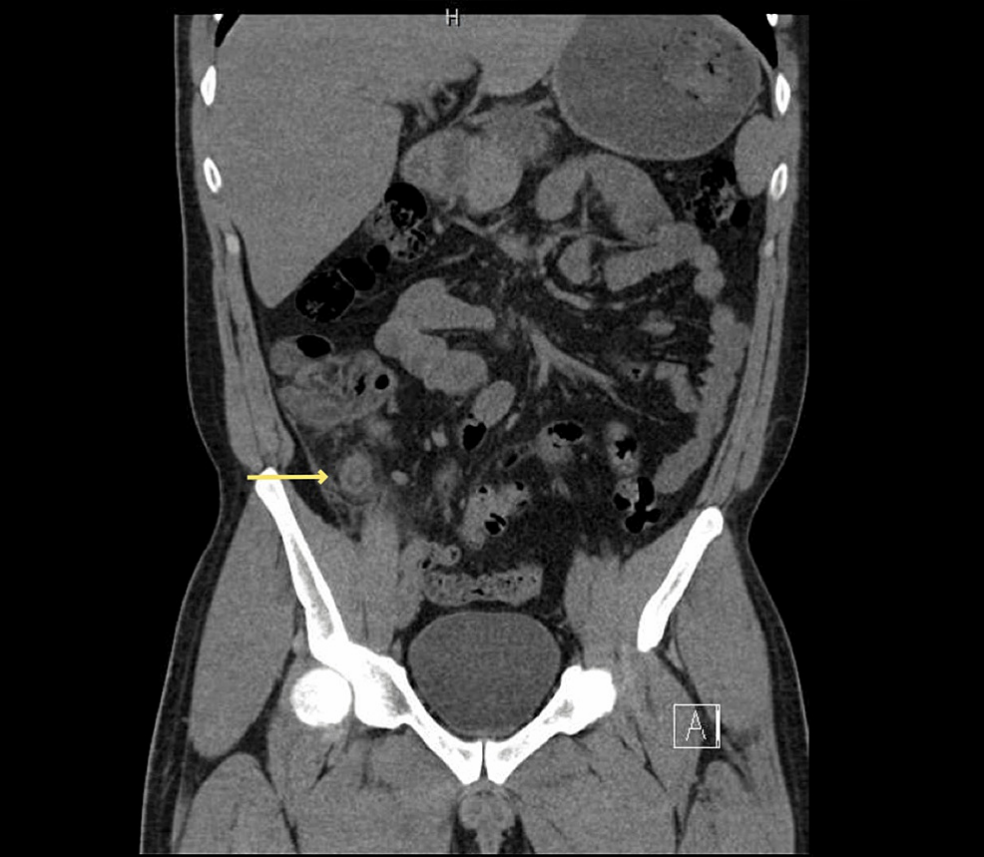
Non-contrast abdominal CT image showing wall thickening of a blind-ended tubular structure arising from the cecal pole (yellow arrow), suggestive of inflamed appendiceal stump.
